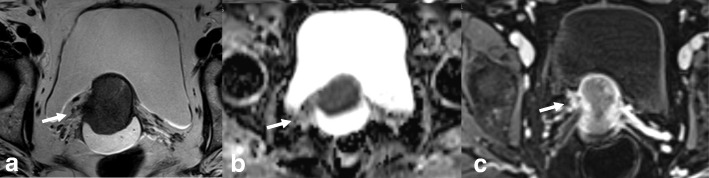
Tumor visualization with axial oblique T2WI (a), DWI-ADC map (b), and DCE imaging (c) reveals loss of the hypointense cervical rim on the right lateral aspect of the cervix, with an irregular interface between the tumor and the parametrium (arrow). ADC map shows diffusion restriction, and DCE imaging reveals enhancement of the right lateral wall indicating early parametrial invasion (arrows)
